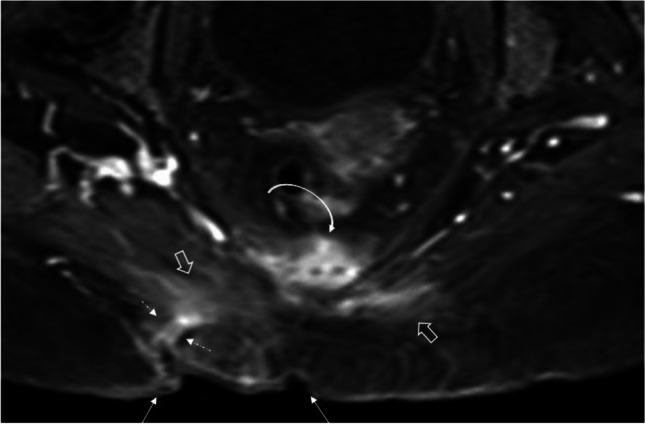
Fat-suppressed postcontrast T1W MR image shows sacral decubitus (arrows) ulcer in a 61-year-old diabetic patient. Note the irregularity of skin and the “tram-track” appearance (dashed arrows) of the sinus tract that opens to the ulcer base. Enhancement of the adjacent gluteus maximus muscles (open arrows) and sacral vertebra (curved arrow) indicate pyomyositis and osteomyelitis, respectively. Tissue cultures revealed polymicrobial etiology of *Acinetobacter baumannii* and *P. aeruginosa*
